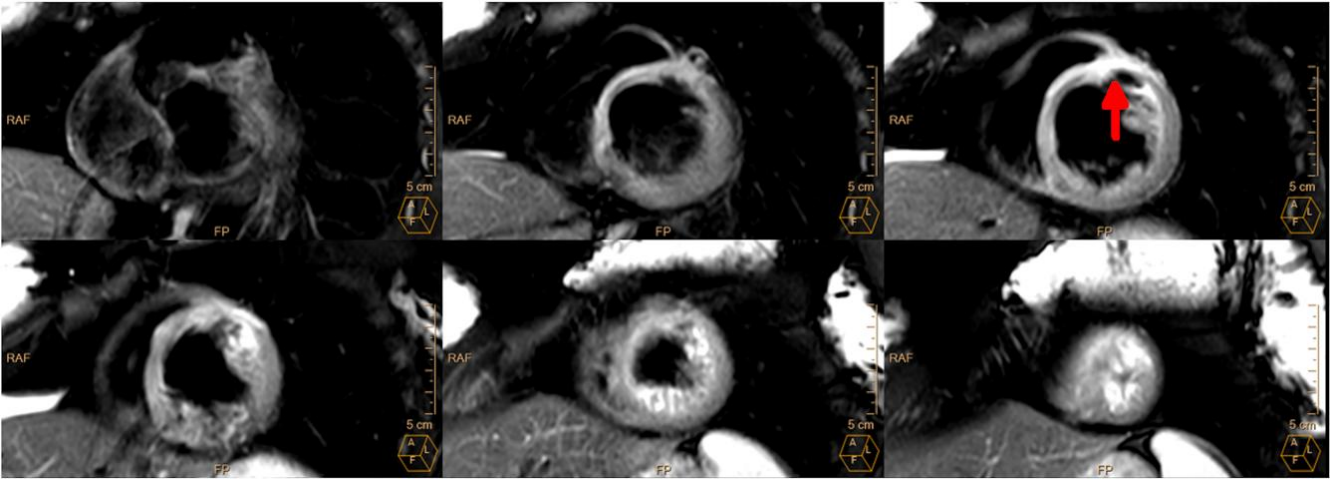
Acute myocardial oedema on cardiac magnetic resonance 3 days after initial presentation. T2-weighted short axis CMR imaging shows acute myocardial oedema in septal and anterior segments, corresponding with LGE imaging.
